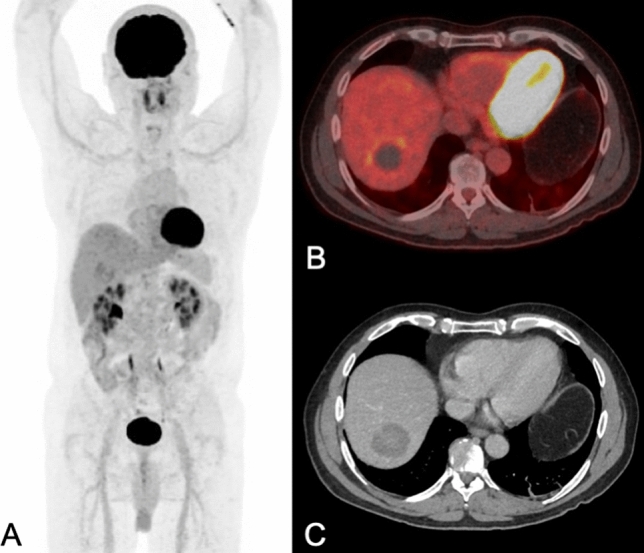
PET/CT performed for staging of alveolar echinococcosis in a 55-year old man (patient 02 in Table 3) showed mild metabolic activity [in maximum intensity reconstructions of PET (A) and fused PET/CT images (B)] at the margins of a round and hypodense lesions in the right liver lobe [as shown on axial contrast-enhanced CT images in (C)]. SUVmax was 4.7 in the echinococcosis manifestations, and 3.2 in non-infected liver tissue; SUVratio was 1.5. After 1000 days of benzimidazole therapy, levels of Em-18 antibodies became undetectable, but therapy was not yet stopped at the end of the present study period (in total after 1131 days of therapy).
